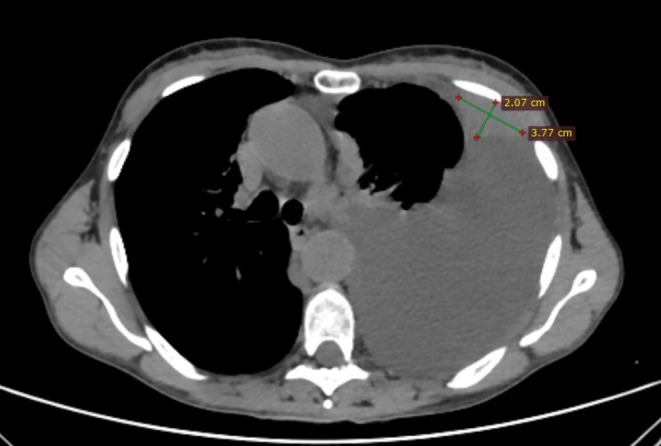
Axial view of a High‐Resolution chest CT scan in the mediastinal window showing a massive left pleural effusion and the largest pleural nodule on the anterior parietal pleura, which underwent ultrasound‐guided percutaneous biopsy.
